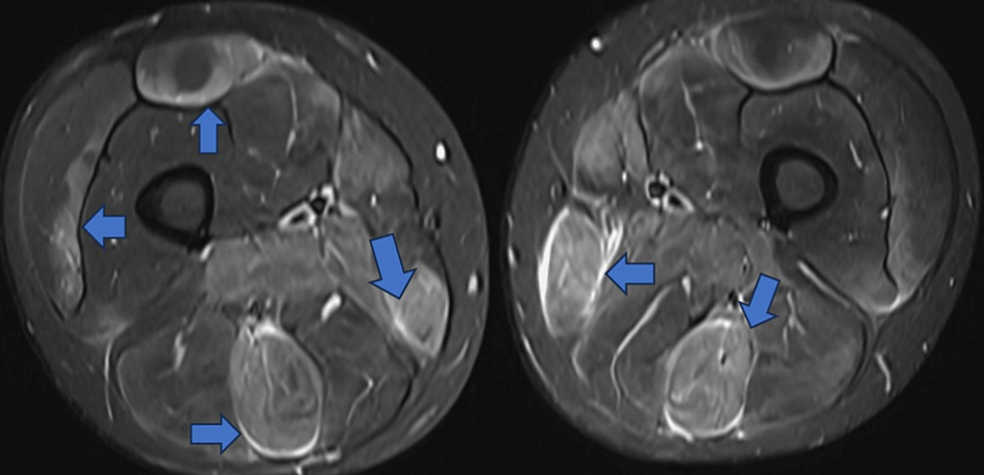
MRI shows areas of high signal intensity, suggestive of acute myositis, indicated by blue arrows.
